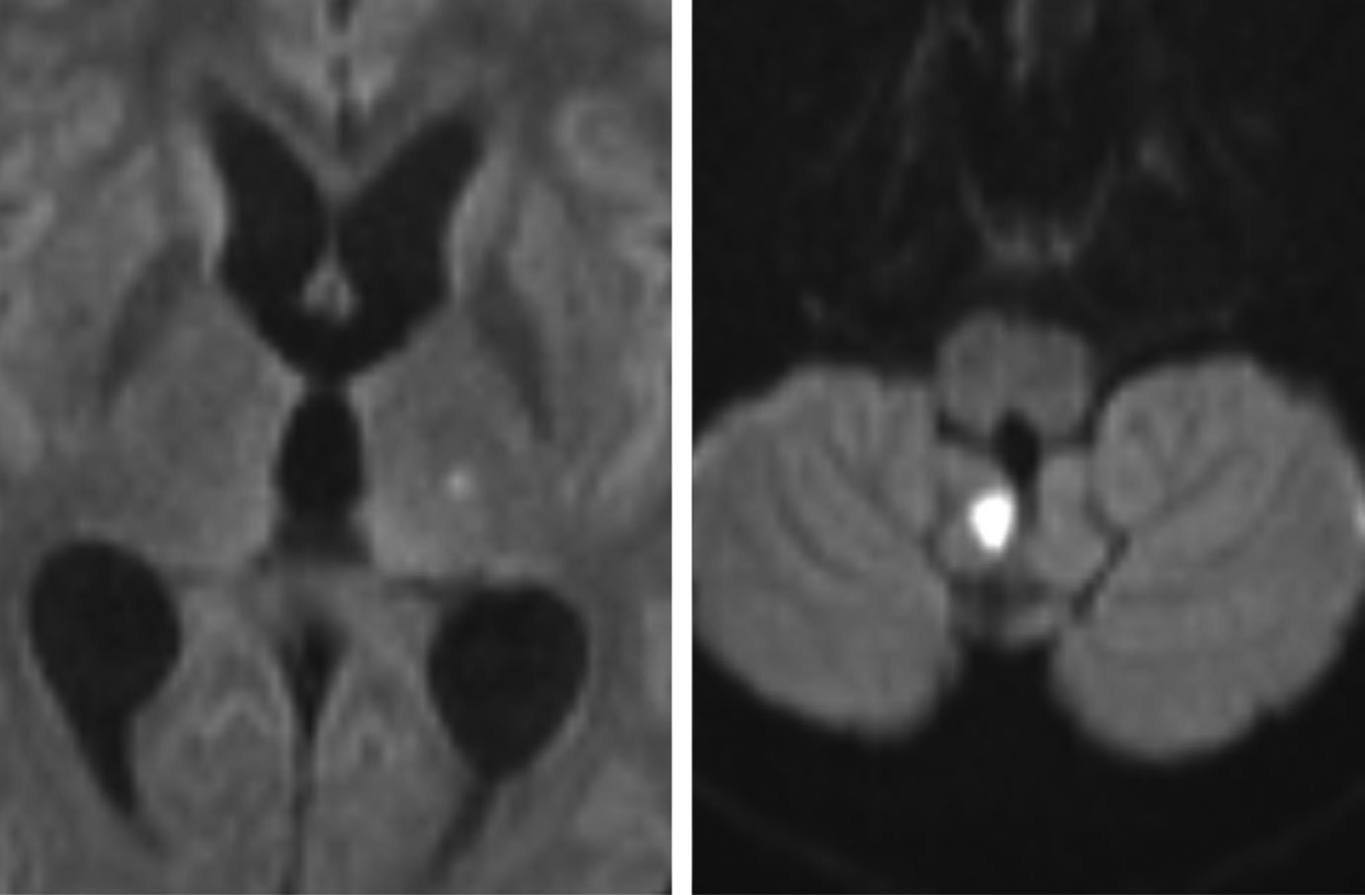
Gadolinium‐enhanced MR images of the head and cervical spine demonstrate dilatation of the cerebral ventricles
